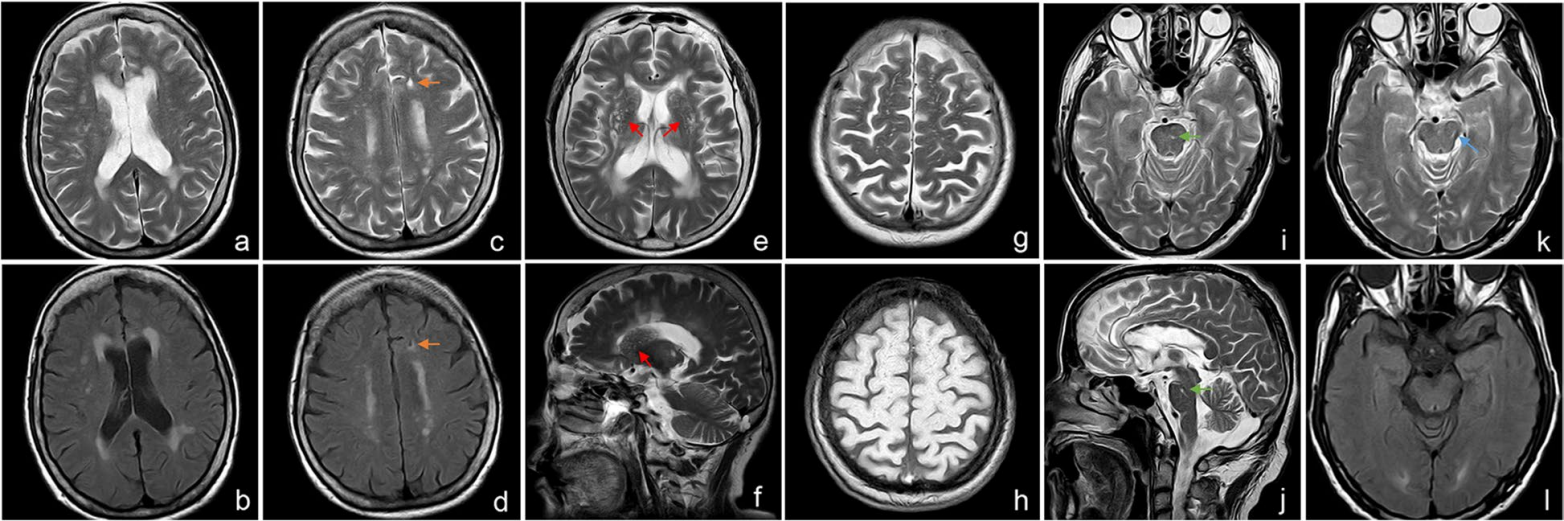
Imaging features of cSVD in IPI patients. (a-d) T2 and FLAIR axial images were from a female patient aged 74 years. (a-b) Moderate WMH with a Fazekas score of 3. (c-d) One lacune of presumed vascular origin located in the left subcortical area (orange arrows). (e-f) T2 axial and sagittal images were from a female patient aged 73 years, showing moderate to severe BG-EPVS with a visual score of 3 (red arrows). (g-h) T2 and T1 axial images were from a 69-year-old female patient, showing moderate to severe CSO-EPVS with a visual score of 3. (i-j) T2 axial and sagittal images were from a 64-year-old male patient, showing brainstem-EPVS in the left medial pons (green arrows). (k-l) T2 and FLAIR axial images were from a male patient aged 61 years, showing brainstem-EPVS in the left lateral midbrain (blue arrow)
